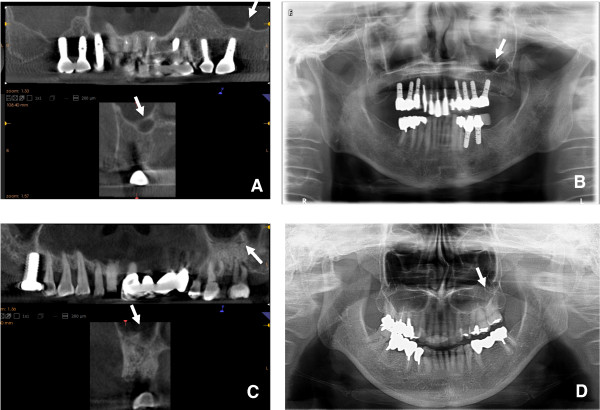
Images of septa in the maxillary sinus. The CBCT (A) and panoramic radiograph (B) of a 69-year-old female with septa >5 mm undergoing preoperative planning for implantation of a left maxillary molar. The CBCT (C) and panoramic radiograph (D) of a 73-year-old female with septa <5 mm undergoing preoperative planning for implantation of a left maxillary molar. The septa in the two patients are clearly visualized on CBCT (A and C). However, the septa >5 mm are visualized on panoramic radiographs (B), but those <5 mm are not (D).
